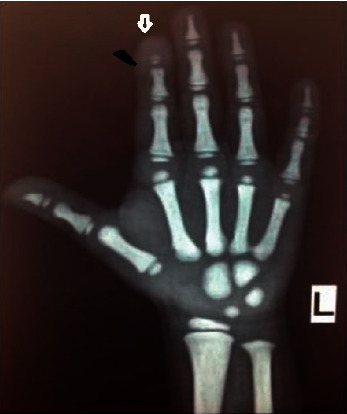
The X-ray of one hand with wrist in case 5-pycnodysostosis patient showing delayed bone age and acroosteolysis in the distal phalanges of thumb and index finger.
